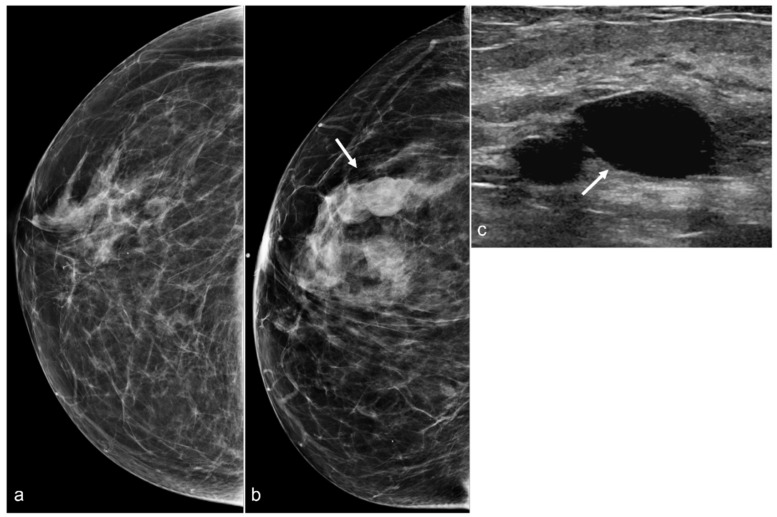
A 58-year-old woman with a history of BIA-ALCL and subsequent BES of retropectoral silicone implants. Post-BES CC mammogram (a) and most recent mammogram CC view (b) demonstrate developing masses (arrow). Right breast ultrasound in the transverse view (c) shows cysts (arrow).
